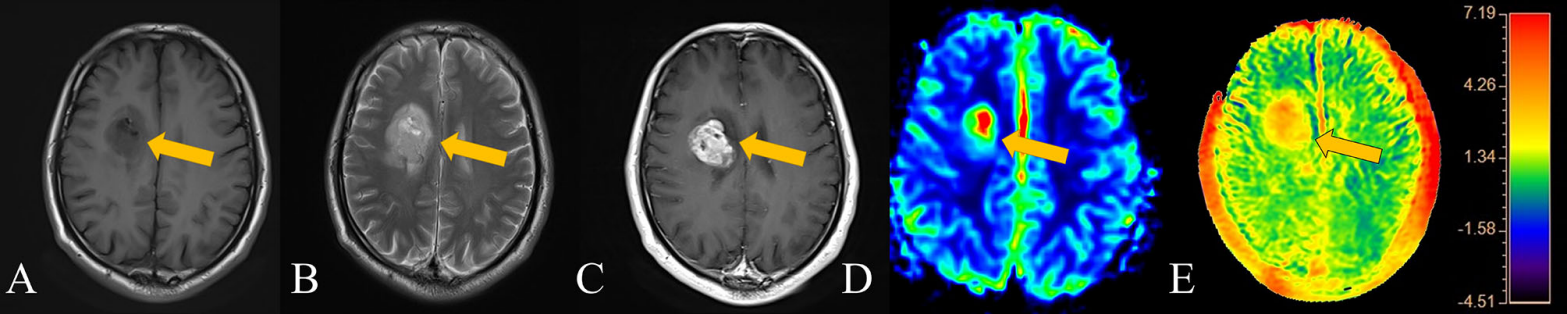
Patient 3, a 62-year-old male, has an HGG in the right basal ganglia. The APT signal range of the patient was obviously larger than that on both T2WI and enhanced scans and larger than that on PWI. (A) T1-weighted imaging showed an iso-slightly low signal. (B) T2-weighted imaging showed an iso-slightly high signal. (C) Enhanced MR imaging showed obvious enhancement. (D) PWI showed marked hyper-perfusion. (E) APT imaging shows that the amide proton transfer rate obviously increased.
